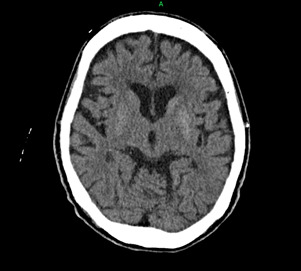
Non-contrast brain CT scan of the patient showing subtle hyperdensity of the left striatum without evidence of mass effect, oedema or volume loss and with no sign of brain ischaemia.
